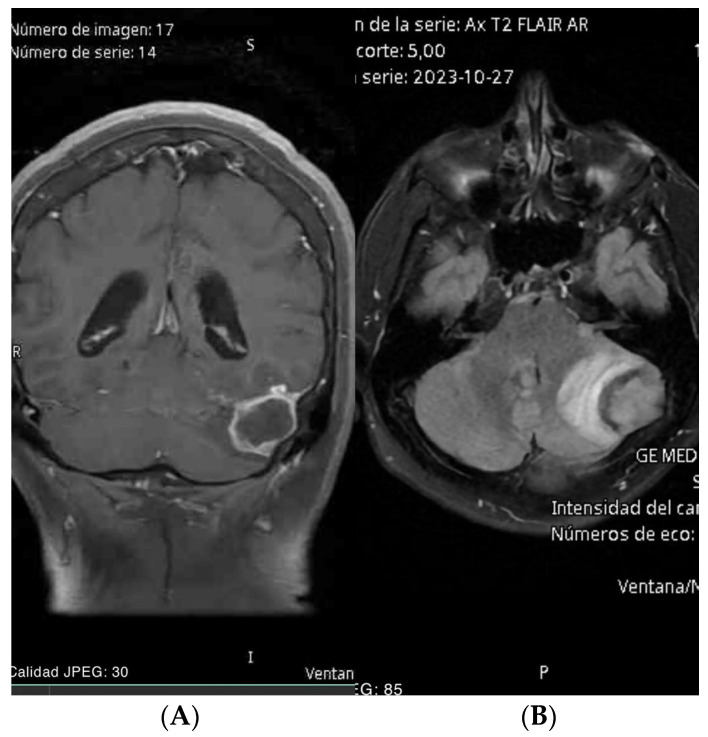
(A) Brain MRI coronal view: extra-axial infratentorial lesion adjacent to the left cerebellar hemisphere with ring enhancement with a volume effect. (B) Brain MRI axial view FLAIR: extra-axial infratentorial lesion adjacent to the left cerebellar hemisphere with a volume effect.
